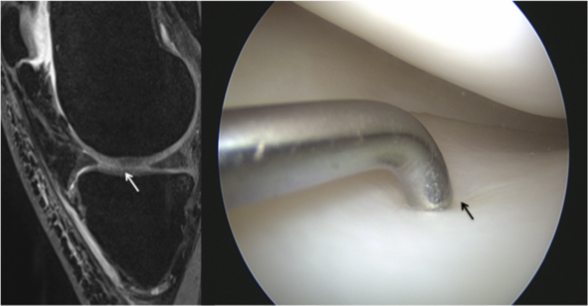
MRI and arthroscopic image of a grade I lesion in an 18-year-old male. The lesion is hardly visible in sagittal source images, coronal reconstructions with a slice thickness of 1.5 mm already miss the grade I lesion
